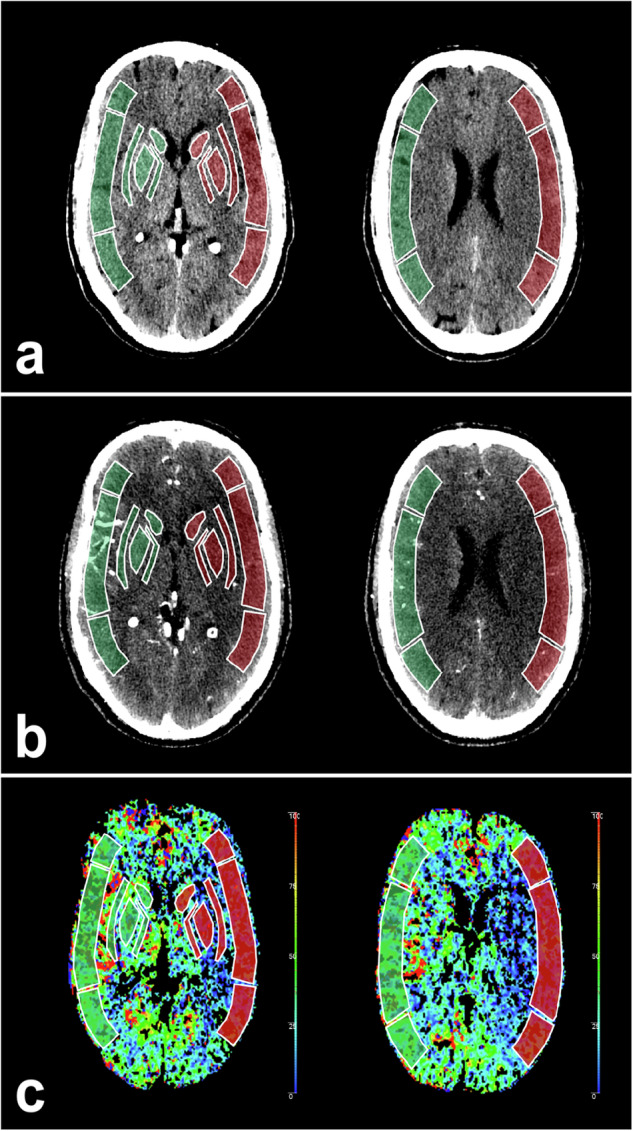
a Initial non-contrast CT (window level/width in HU = 30/40). b CTA (window level /width in HU = 60/90). c CT perfusion-derived CBF map (scale bar added with mL/100 g/min CBF) of an individual with acute infarction in the cerebral territory of the left MCA. The ASPECTS regions on both the affected side (red) and the non-affected side (green) are marked in the different imaging modalities. ASPECTS, Alberta stroke program early computed tomography score; CT, Computed tomography
